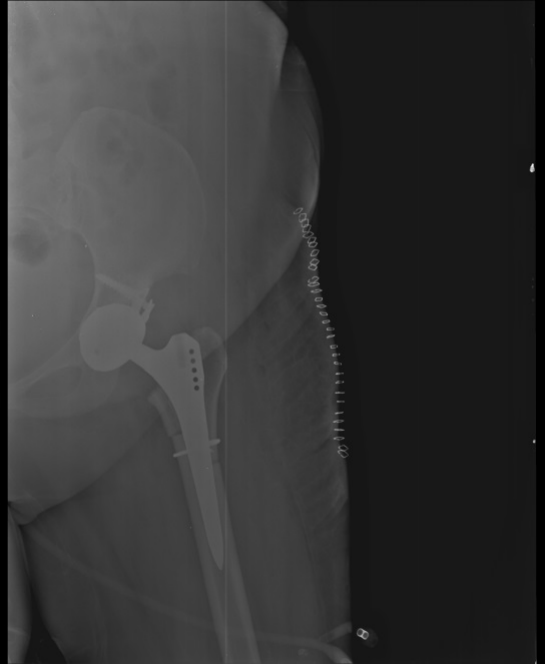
Postoperative X-ray radiograph of patient who underwent osteotomy fixation with only a cable.
